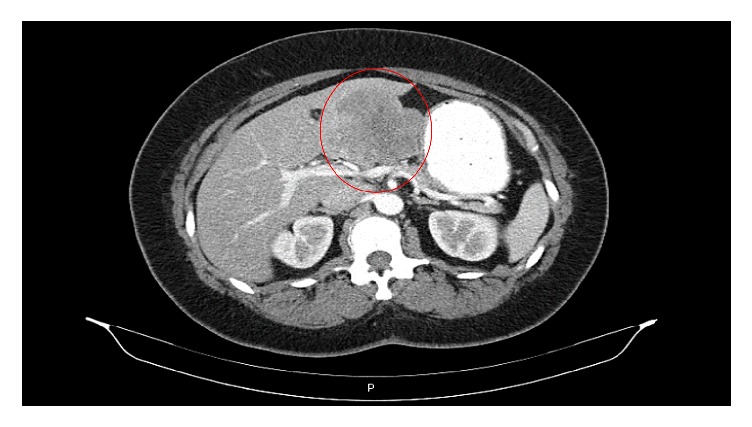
Contrast enhanced computed tomography scan. The image demonstrates a large left hypoattenuating hepatic lobe mass measuring 7.8 × 7.8 cm.
